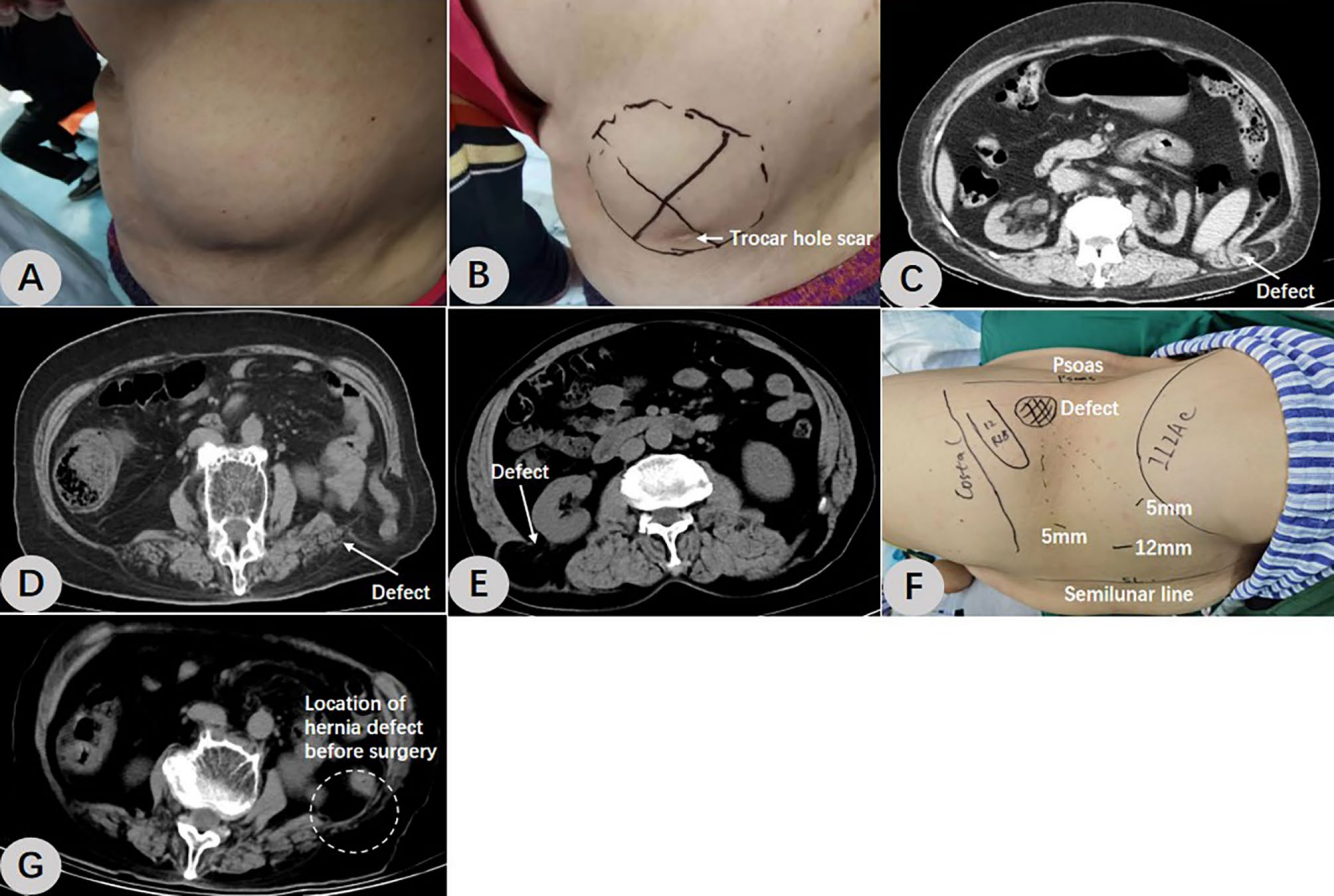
Patients’ signs, CT images, and preoperative surgical markers. (A) A patient with a primary left-side Grynfeltt hernia. (B) A patient with a secondary left-side lumbar hernia. (C) CT revealed a left-side Grynfeltt hernia. (D) CT revealed a secondary left-side lumbar hernia. (E) CT revealed a right-side Grynfeltt hernia. (F) The patient was positioned in the healthy lateral decubitus position with waist hyperextension. (G) The follow-up CT image of the second patient.
